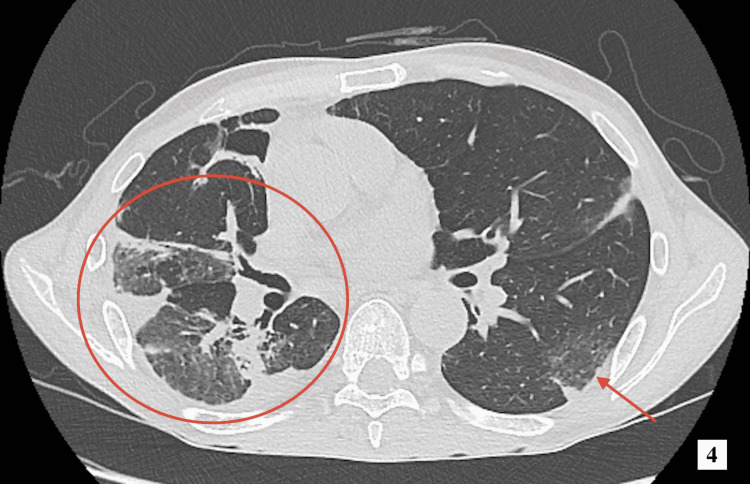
Computed tomography scan of the thorax showing improvement of bilateral opacification and traction bronchiectasis.
